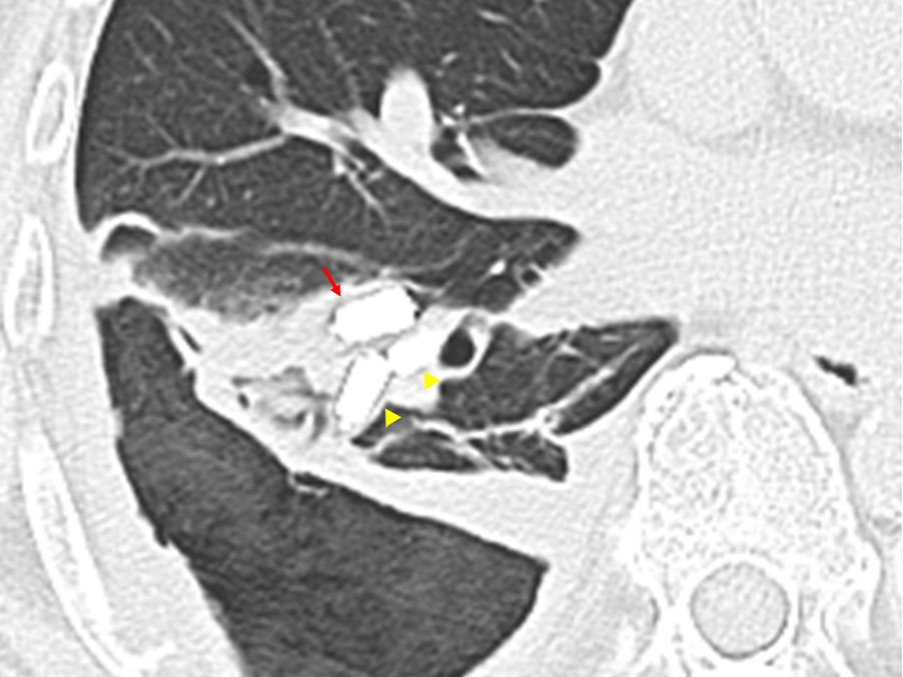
Computed tomography of the chest after the endobronchial occlusion showing appropriate deployment of silicone spigots at right B8 (arrow) and B9 (arrowheads) and the absence of the bronchial fistulas.
